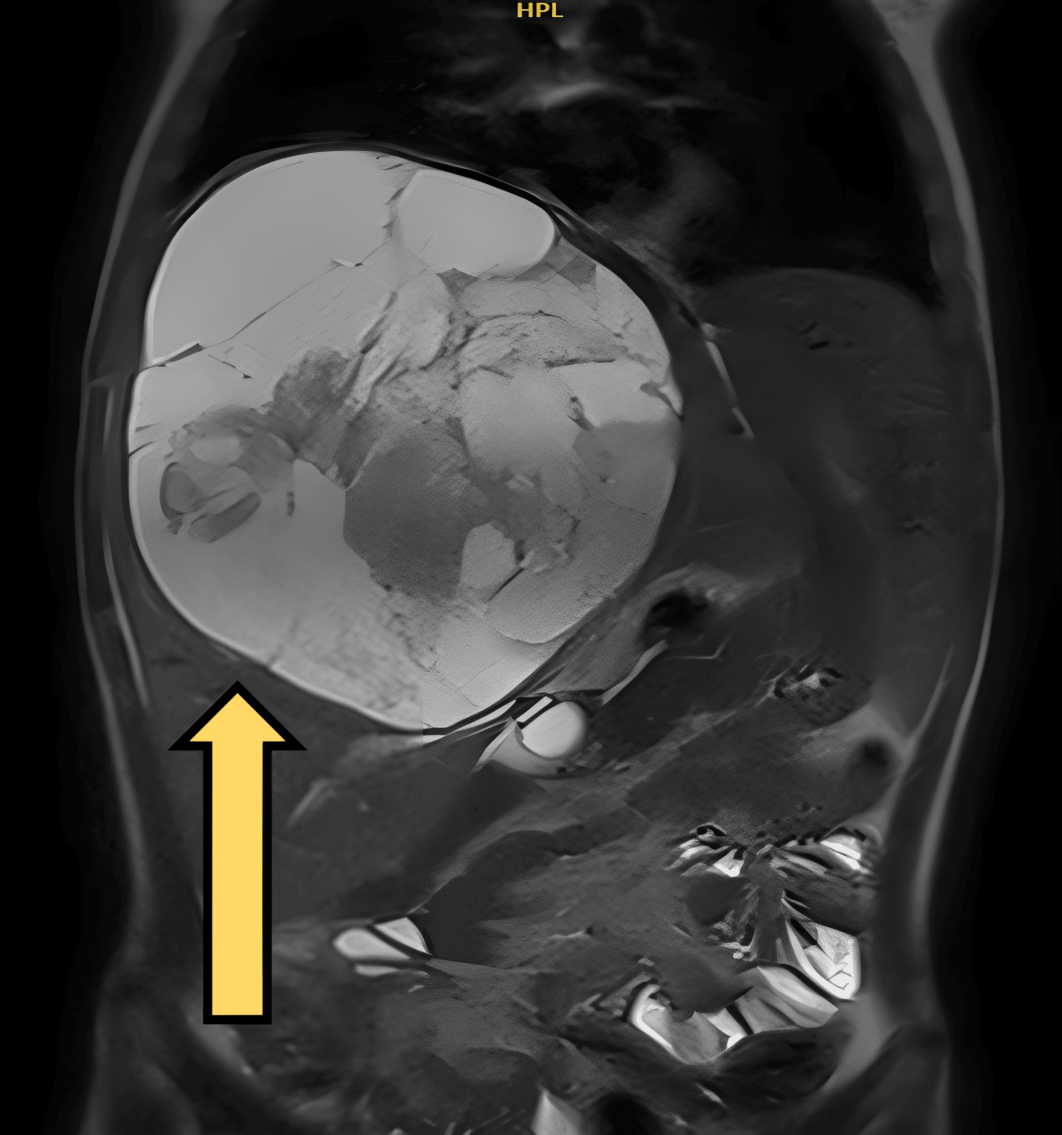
Coronal view of MRI abdomen showing well-defined encapsulated mass of mixed signal intensities measuring approximately 17.6 × 14.3 × 15 cm
